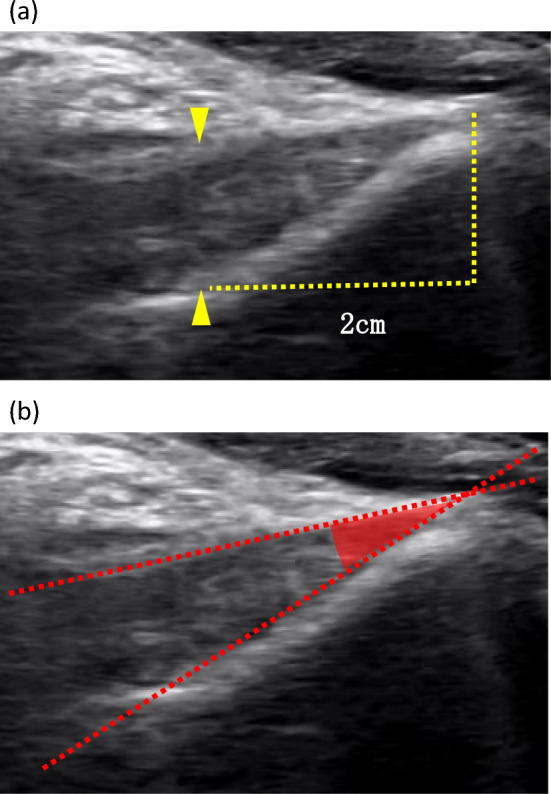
Ultrasonographic measurement method of the posterior cruciate ligament. a The thickness of the posterior cruciate ligament was measured at 2 cm proximal to its insertion into the tibia (between the yellow arrowheads). b The angle of the posterior cruciate ligament was measured using a tangent line drawn from the tibial insertion
